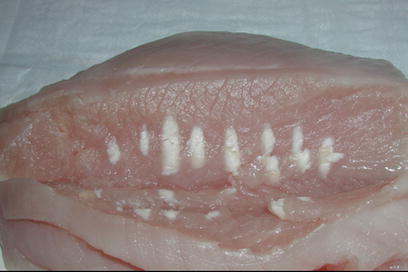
The typical elliptical shape of coagulated tissue after focussed ultrasound ablation
